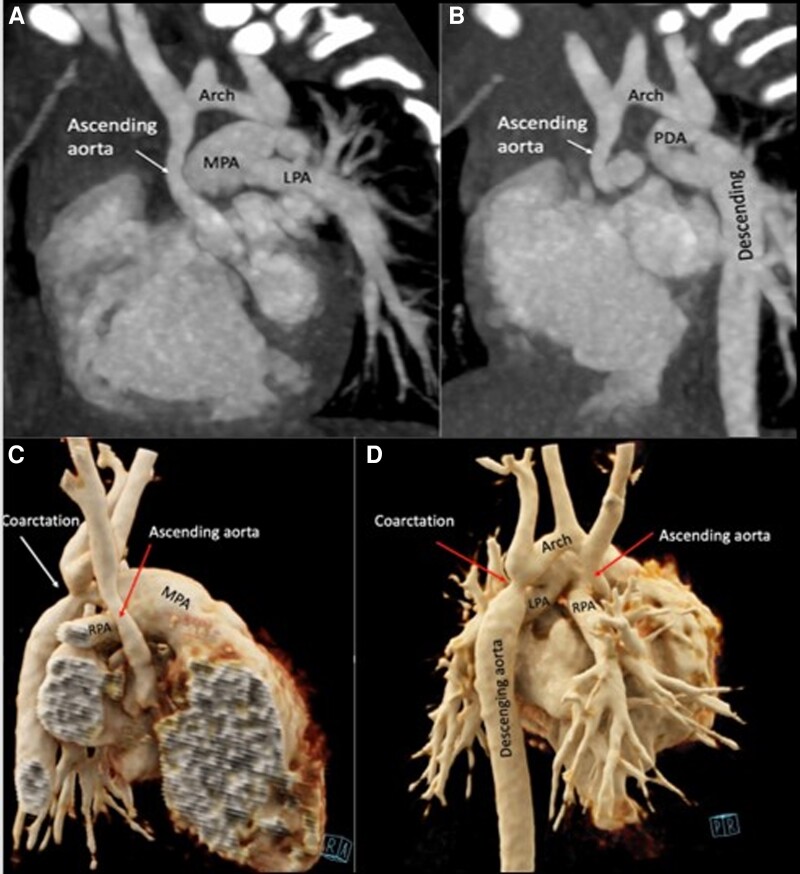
(*A* and *B*) Sagittal oblique reformatted maximum intensity projection image from cardiac computed tomography angiography displays the ascending aorta and aortic arch with pre-ductal coarctation associated with hypoplastic left ventricle syndrome. (*C*, *D*) Three-dimensional volume-rendered image in lateral and posterior views demonstrates diffuse hypoplasia of the aortic root, ascending aorta, and aortic arch.
